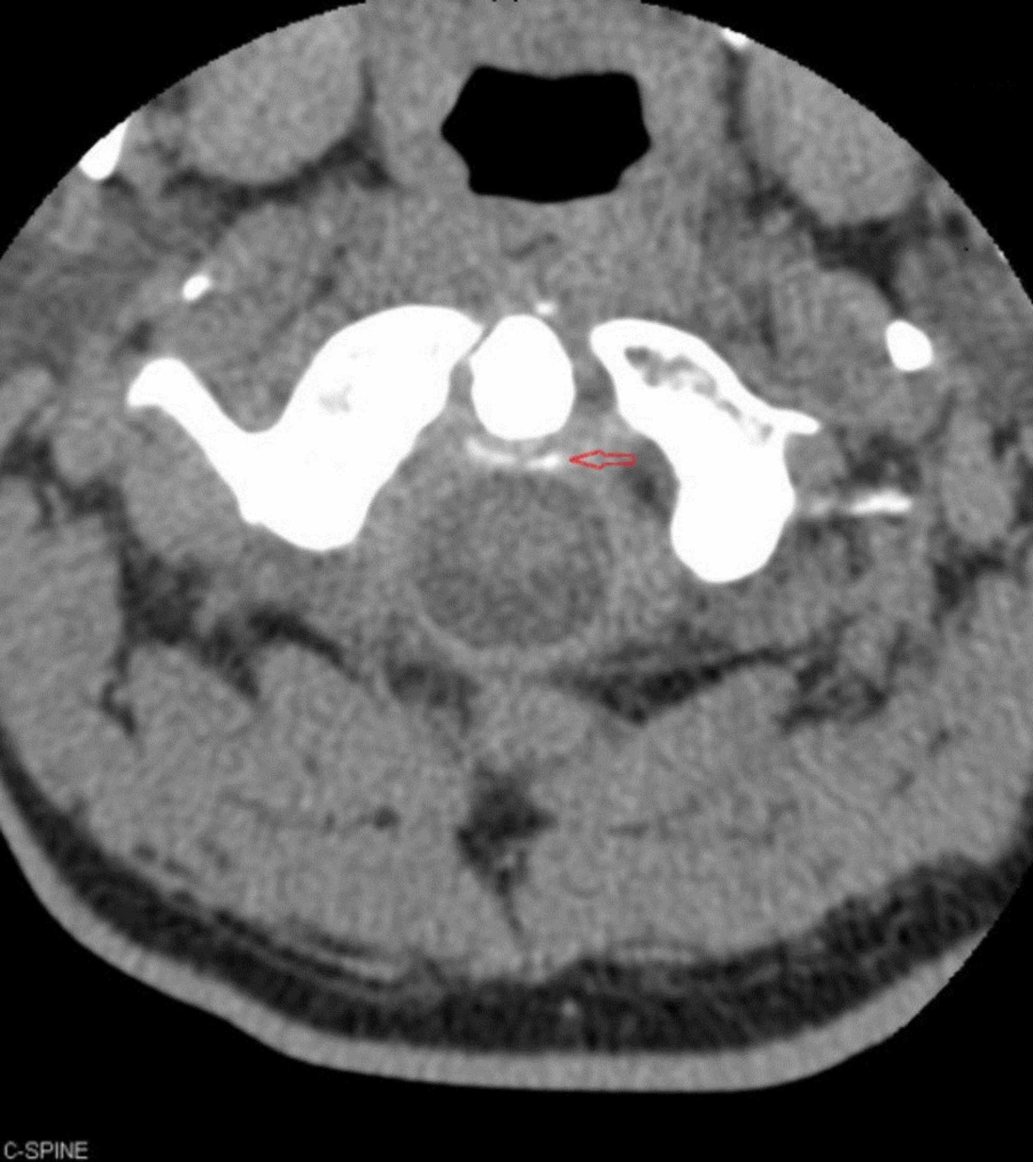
Axial section CT of the neck showing chondrocalcinosis of the transverse ligament of the atlas The arrow highlights area of calcinosis of the transverse ligament posterior to the dens.
